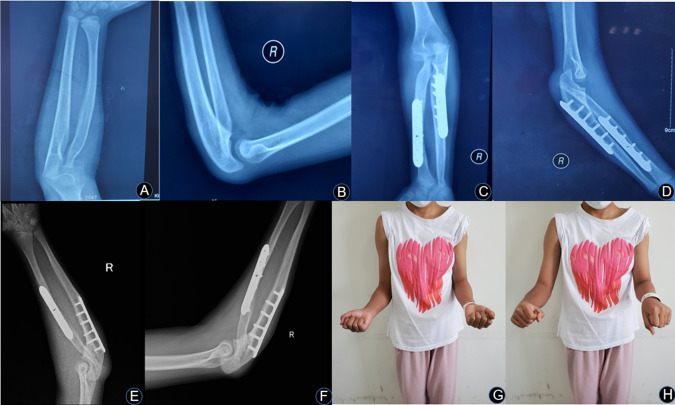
(A,B) A 7-year-old girl who had right proximal radioulnar synostosis that was fixed in the pronation position. Her preoperative Failla score was 8 points. Preoperative anteroposterior and lateral views of the right proximal radioulnar synostosis show a Cleary and Omer type III deformity. (C,D) Bone union was achieved at the osteotomy site after 3 months of surgery. (E,F) Excellent bone result is shown on anteroposterior and lateral views of X-ray at 7 years after the operation. (G,H) The rotational function of the right forearm was significantly improved at the last visit. The patient’s daily activities were greatly improved.
